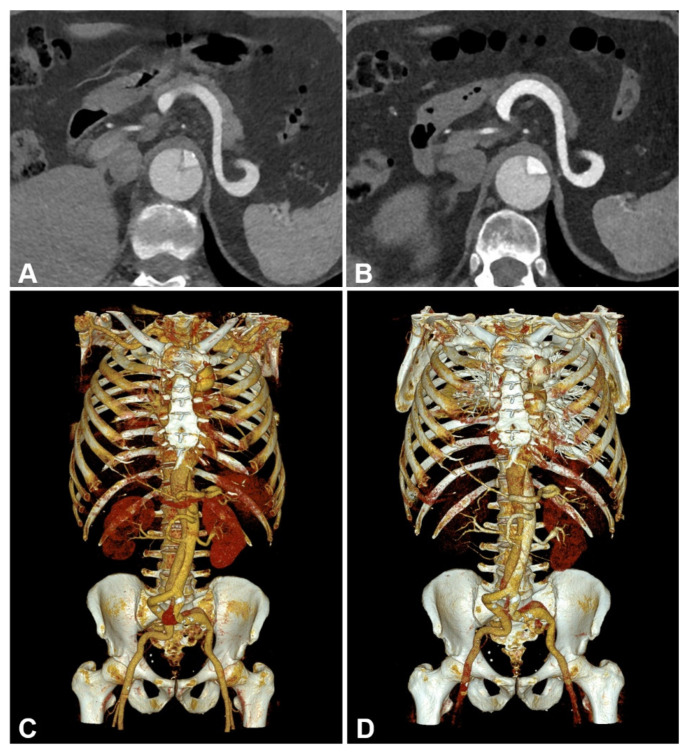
Side-by-side comparison between energy-integrating-detector computed tomography (EID-CT) (A,C) and photon-counting-detector CT (PCD-CT) angiography (B,D) in a 61-year-old man with surgically repaired aortic dissection. EID-CT: CTDI_Vol_ 5.93 mGy; SSDE 6.52 mGy. PCD-CT: CTDI_Vol_ 4.58 mGy; SSDE 5.26 mGy. (A,B) representative axial orientated CTA images (reconstruction kernel Bv36; slice thickness/increment 3 mm; window setting: width 700 HU/center 100 HU) (C,D) cinematic volume rendering images.
